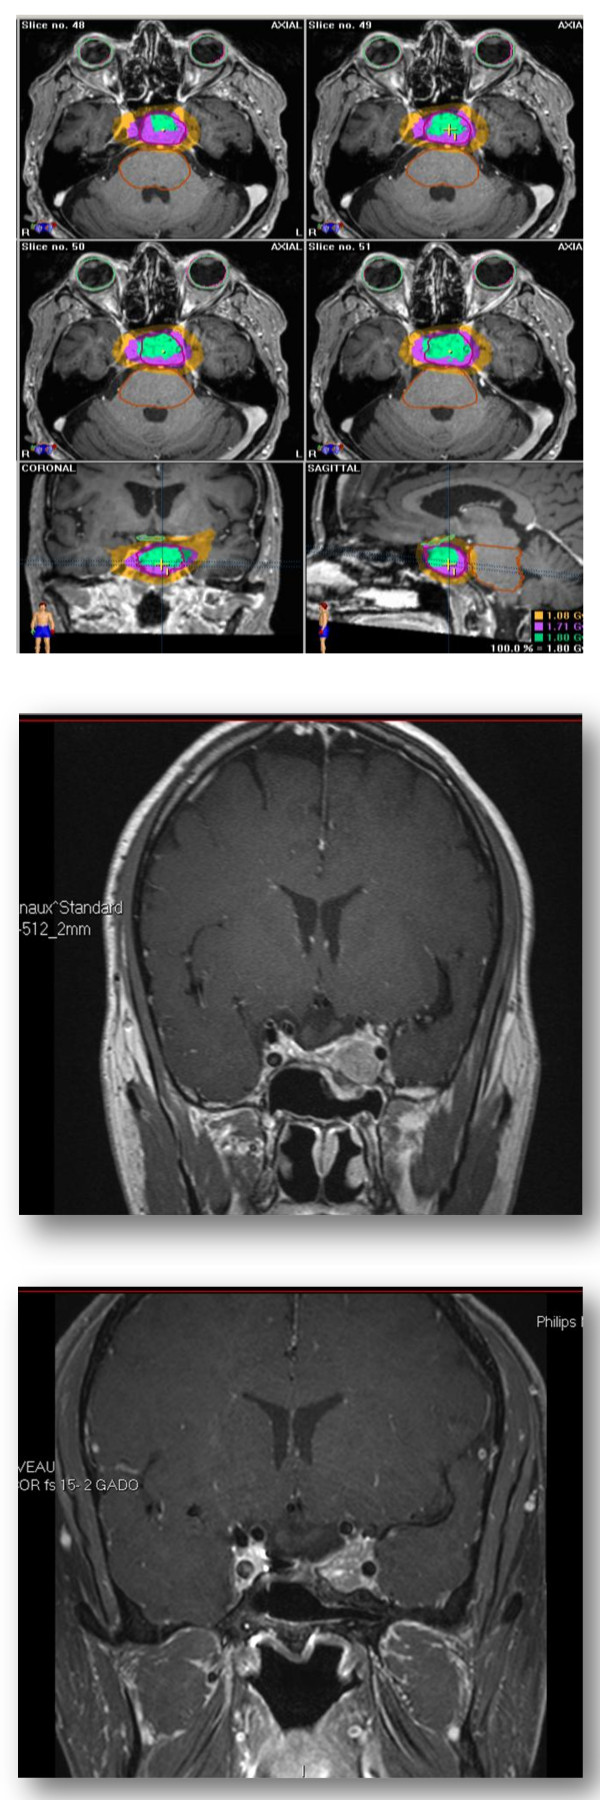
Pre- and post-treatment MRI and CT scan with the dose deposition.
